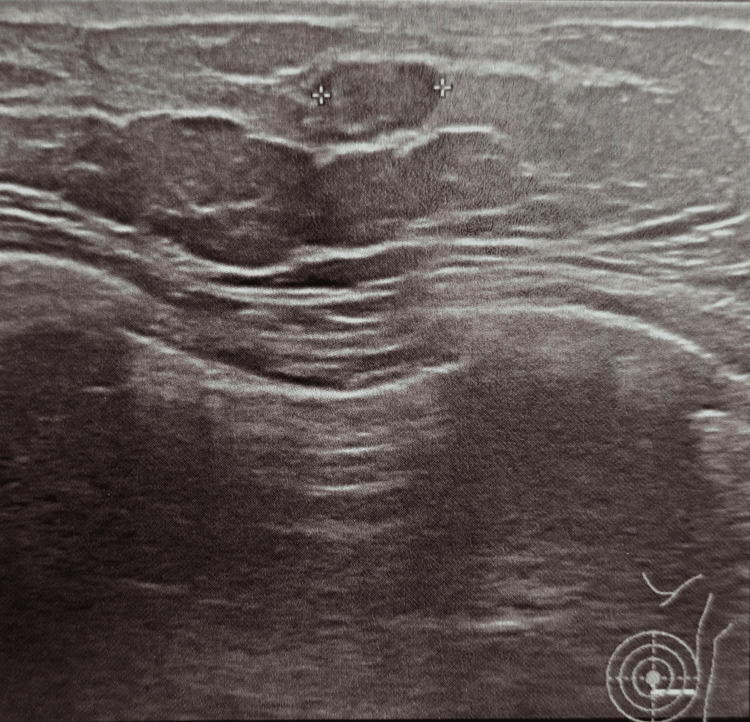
Breast ultrasound showing the hypoechoic nodule which has increased in size from 7.5 mm to 11.6 mm on the left breast
